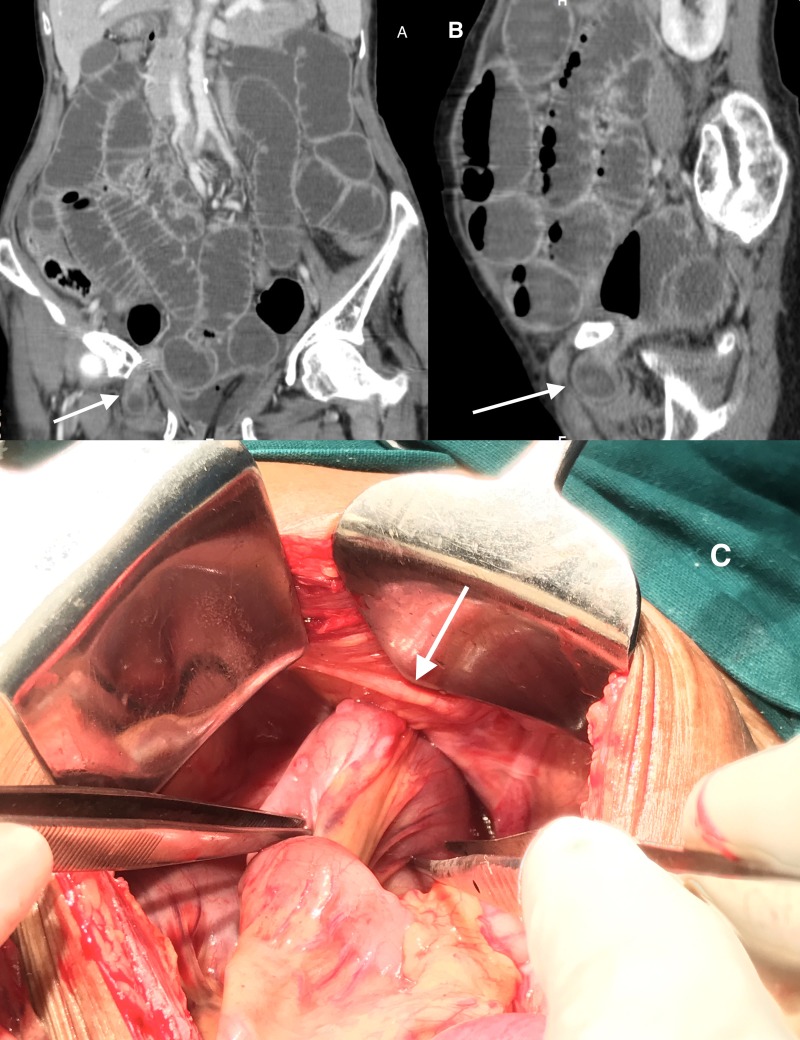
Coronal and sagittal view of CECT image showing small bowel loops entering the right obturator foramen and lying between the pectnieus and obturator externus muscle (arrow). Intraoperative image showing ileal loops entering the right obturator foramen (arrow). CECT: contrast-enhanced computed tomography
